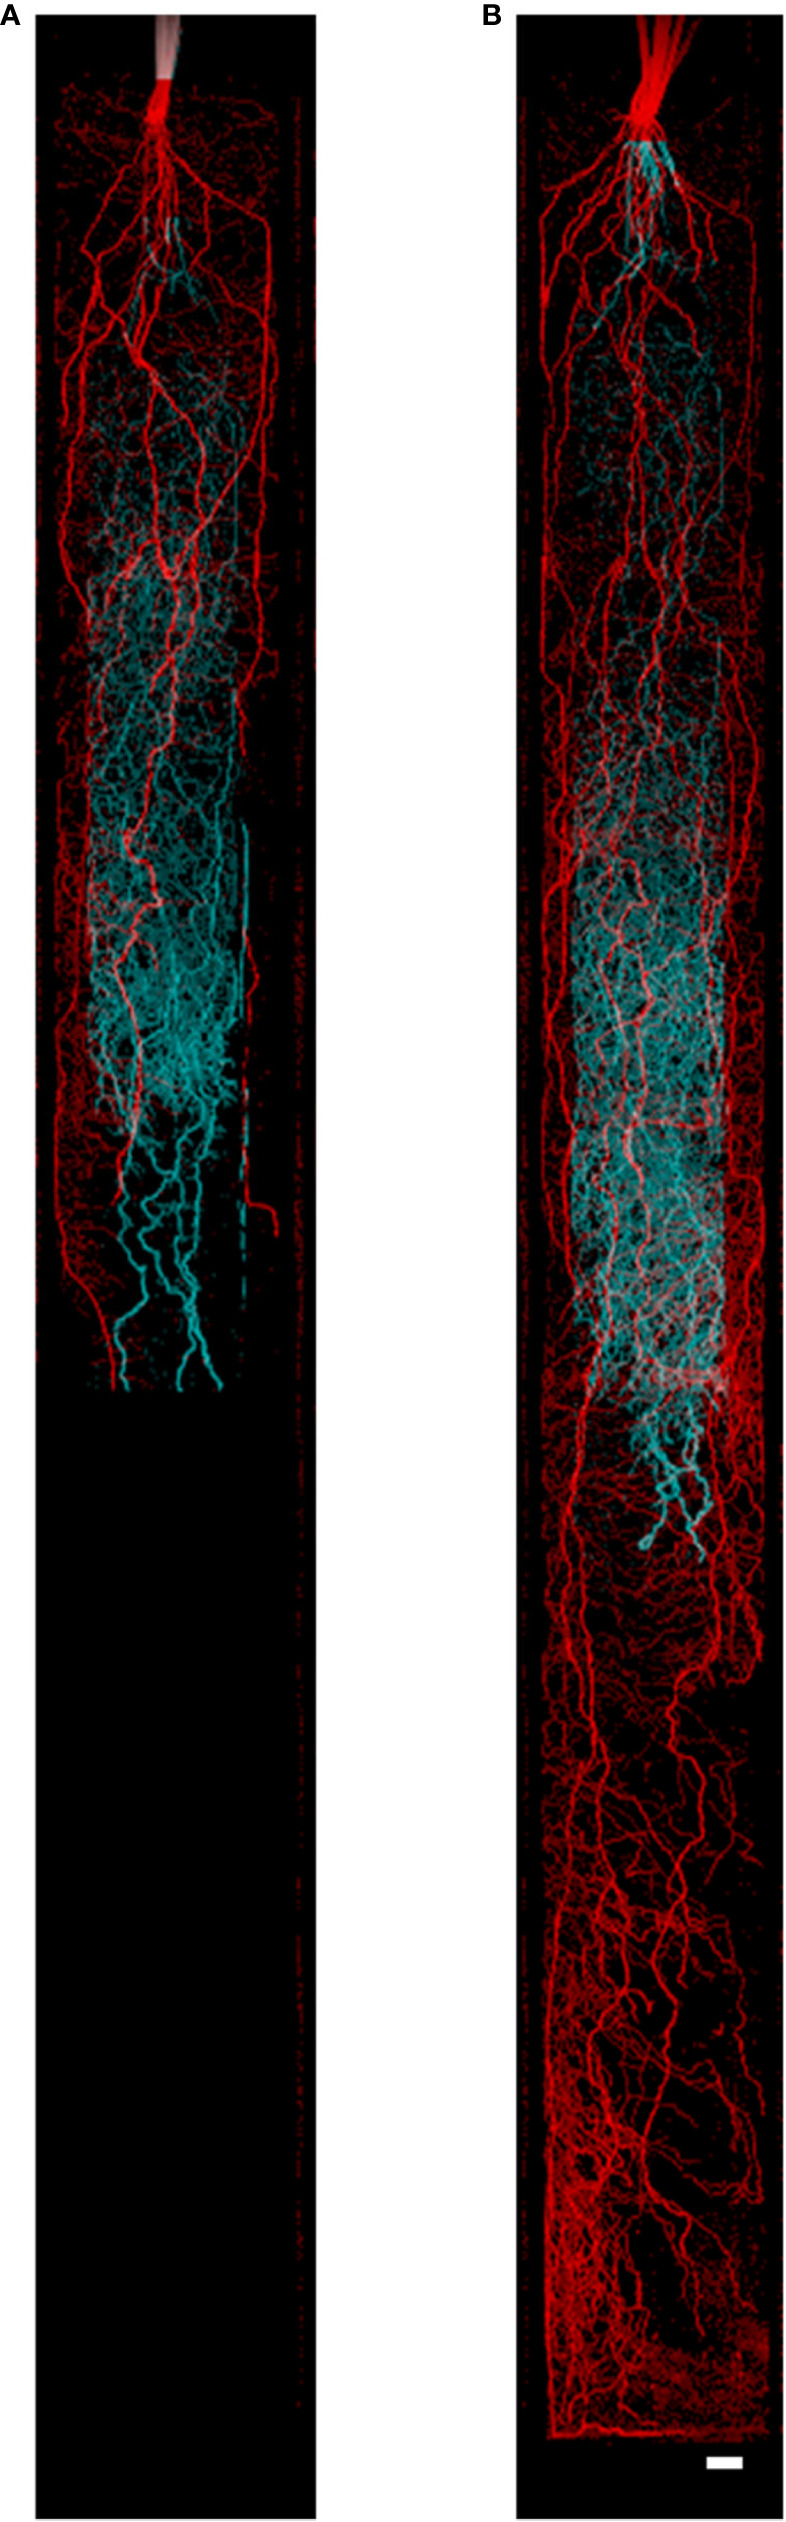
Nodal and seminal root systems as acquired by MRI at (A) 34 days after germination (DAG) and (B) 43 DAG. The seminal root system is represented in cyan growing in the inner cylinder, and the nodal root system is represented in red growing in the space between the inner and outer cylinder. Image (A) consists of five slice blocks of 96 slices (resolution 0.5 × 0.5 × 1 mm^3^), whereas (B) consists of nine slice blocks. Each slice block required 20 min of measurement time. To separate the seminal and nodal root systems, masks were constructed with the same size as the physical compartments. Plants were grown in 80 cm mesocosm. Scale bar = 1.5 cm.
